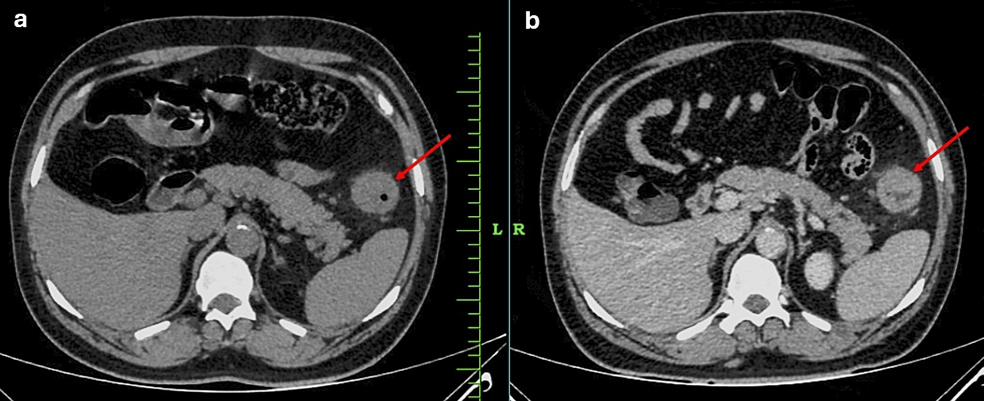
CT (a) plain and (b) contrast-enhanced (venous phase) images of the abdomen showing heterogeneously enhancing asymmetrical circumferential irregular wall thickening causing severe luminal narrowing involving splenic flexure (red arrows) with periserosal fat stranding.
